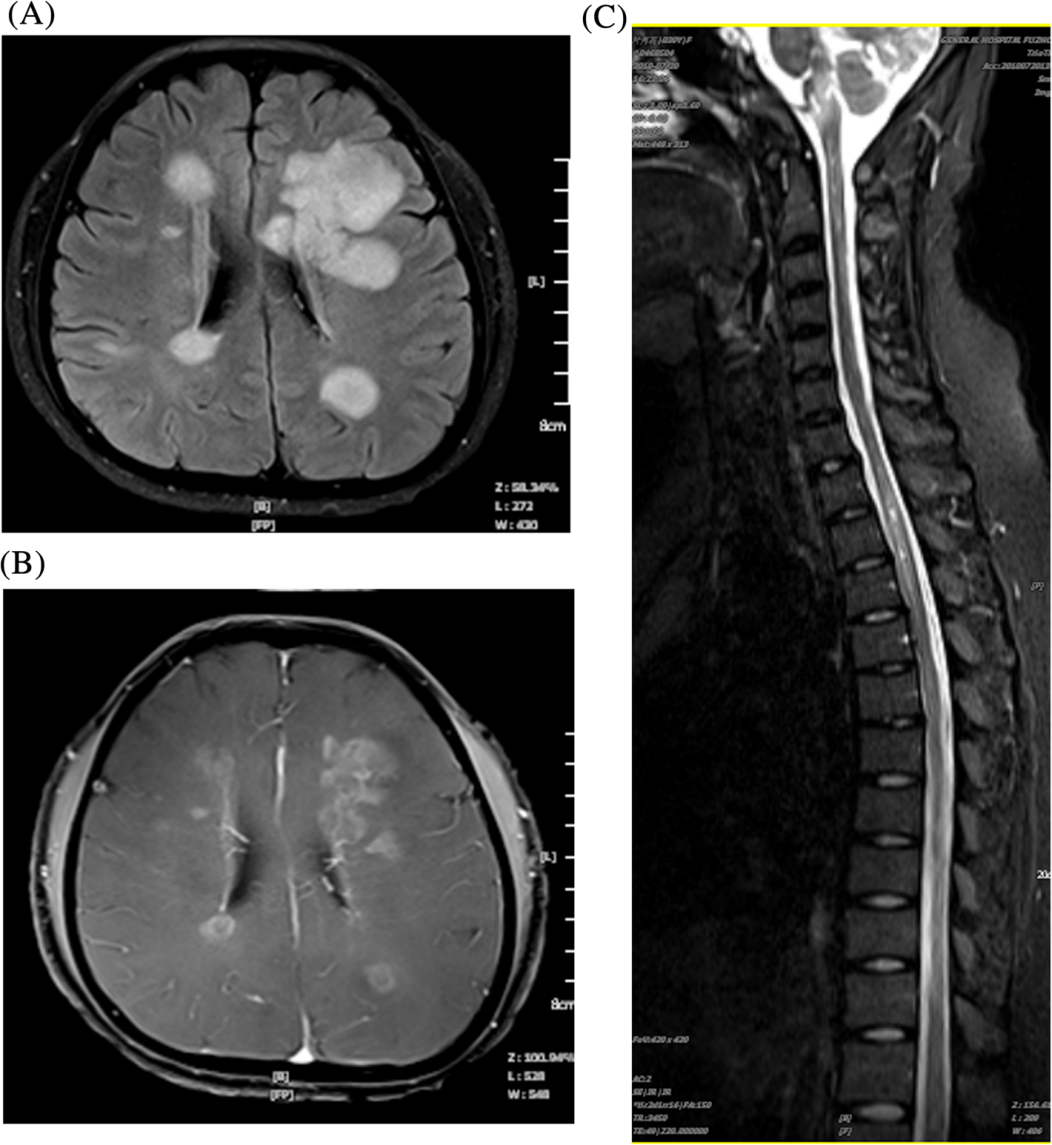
Magnetic resonance imaging (MRI) scans from a 31-year-old male patient with conventional multiple sclerosis who presented with slow reaction times and memory loss for three days. The patient had 97% small lymphocytes in the cerebrospinal fluid (CSF) and was oligoclonal band positive. (A) Periventricular lesions manifested hyperintense in fluid-attenuated inversion recovery (FLAIR) sequence. (B) Enhanced MRI T1-weighted image showed uniform nodular, circular lesions. (C) The lesions were also concentrated in the cervical and thoracic spinal cord, and were distributed along the long axis of the spinal cord and fat-suppressed T2-weighted image remained hyperintense.
